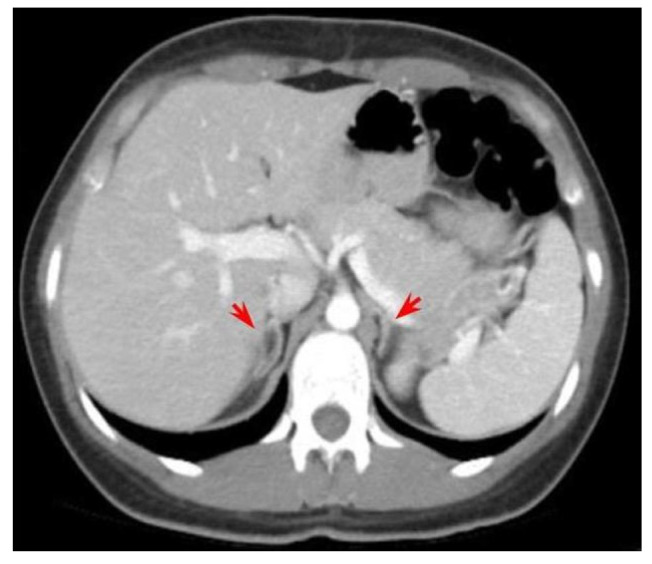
Bilateral adrenal hyperplasia (red arrows) according to computed tomography exam on a prior unreported case of a 26-year-old female diagnosed with HSD3B2 deficiency and SV-CAH form (no tumour was suspected in this case since the imaging aspect is rather usual in this situation).
